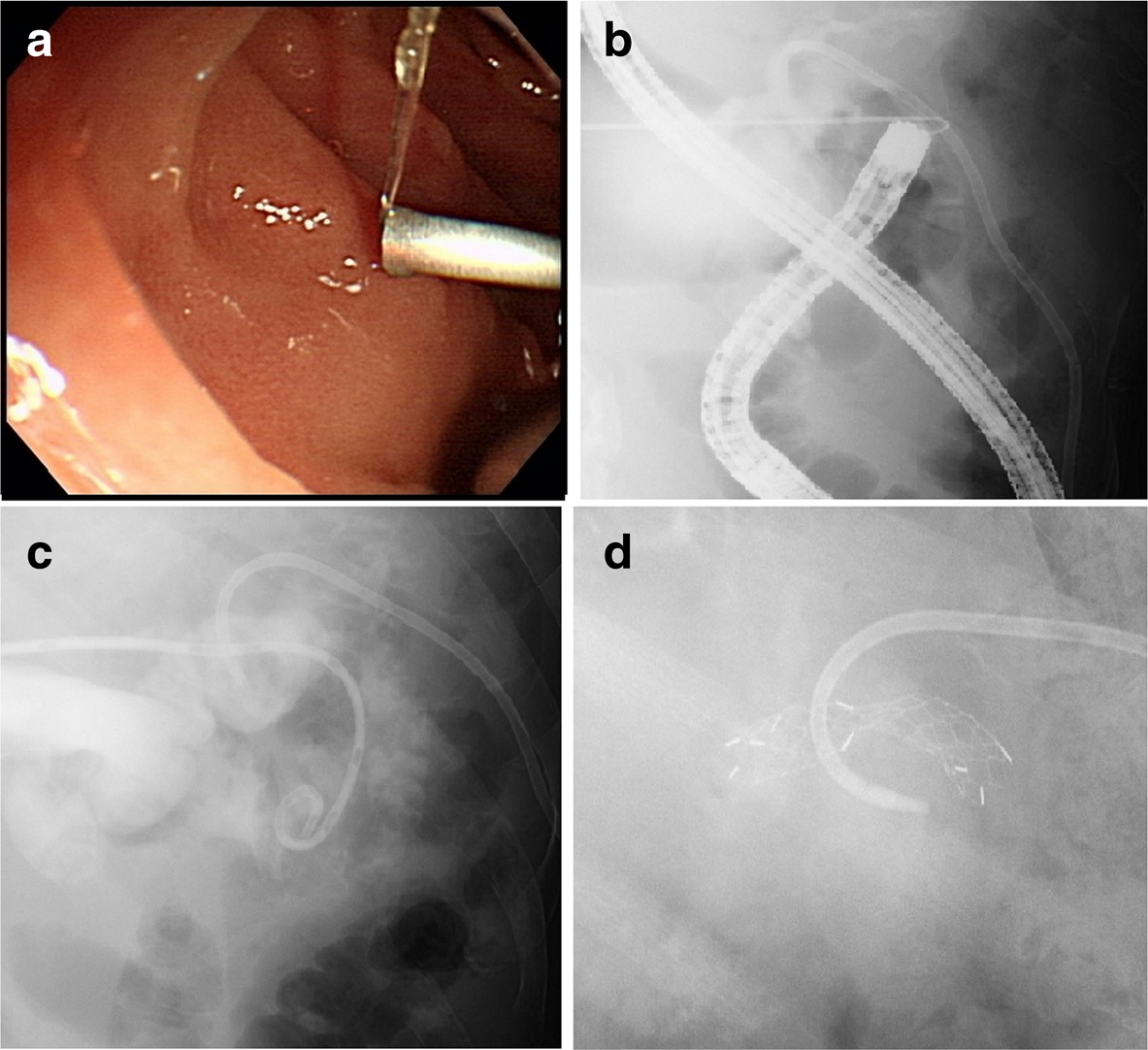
a Puncture needle penetration into elevated jejunum. b X-ray at the time of penetration of puncturing needle into the elevated jejunum. c 8.5-Fr pigtail catheter was inserted into the elevated jejunum. d Uncovered metallic stent (5 cm 10 mm) was inserted percutaneously
